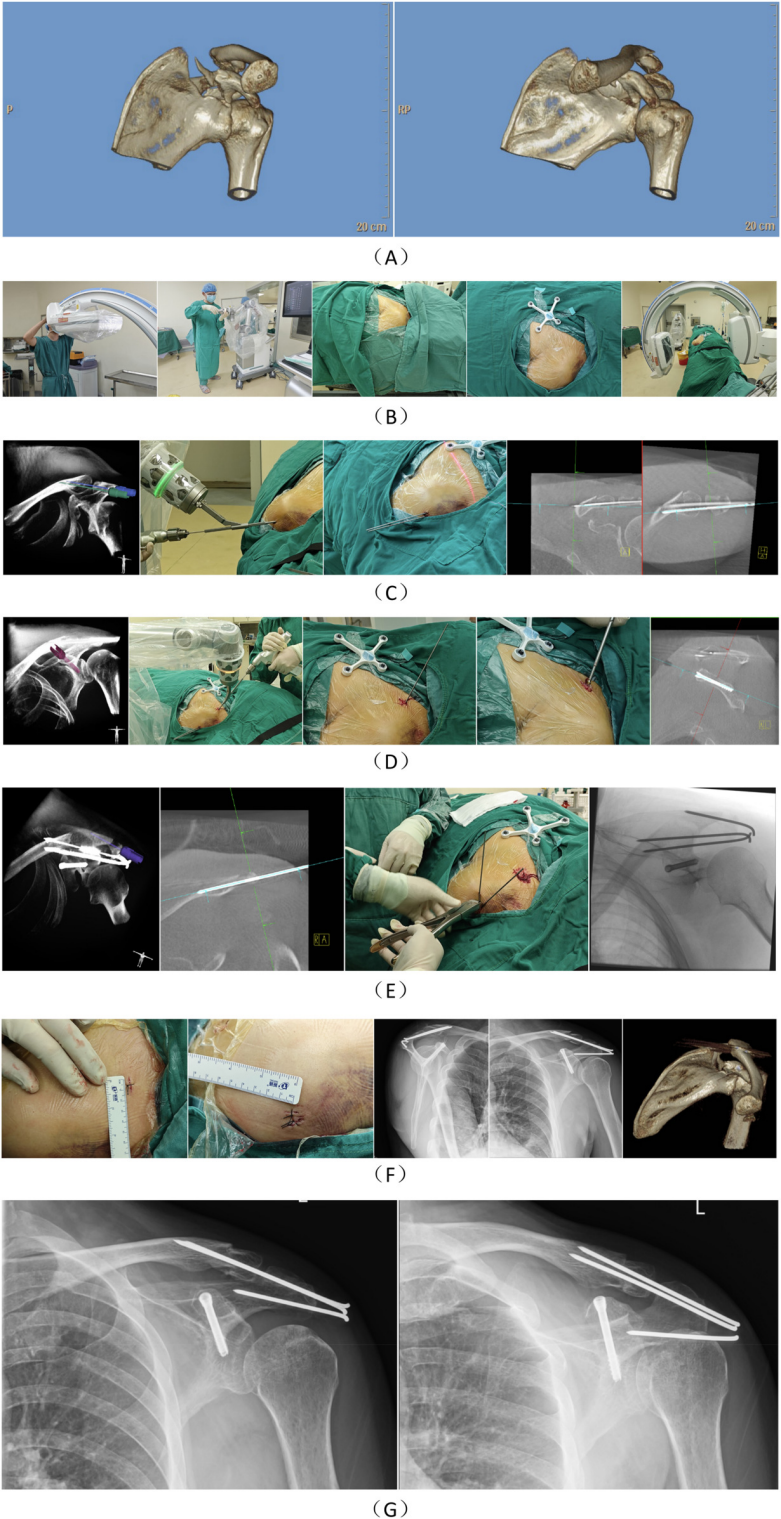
Case presentation: A 54-year-old male patient sustained a fracture of the left scapular coracoid process due to an accidental fall, accompanied by a left clavicle fracture, fractures of the left 2nd to 8th ribs, and pulmonary contusion. (A) The fracture morphology of the patient was characterized by a coracoid base fracture extending into the scapula. (B) A sterile working environment was established for the robotic arm and the image intensifier of the C-arm x-ray machine using a disposable sterile protective cover. After routine disinfection and draping, the patient tracker was installed. Three-dimensional imaging data of the surgical area were collected and uploaded to the robotic surgical planning platform. (C) The surgical paths for two Kirschner wires were first planned, and the Kirschner wires were implanted with robotic assistance. The primary objective of this step was to initially reduce and fix the clavicle to prevent the coracoclavicular ligament from interfering with the reduction of the coracoid fracture. (D) The coracoid screw was planned, and a guide wire was implanted with robotic assistance, followed by the insertion of the screw along the guide wire. (E) Another Kirschner wire was planned to fix the acromion. After completing all internal fixations of the fractures, the Kirschner wires were bent and cut. (F) The patient's postoperative incision condition and postoperative imaging were documented. (G) The patient's x-ray images at 4 months postoperatively showed that the fracture had healed.
